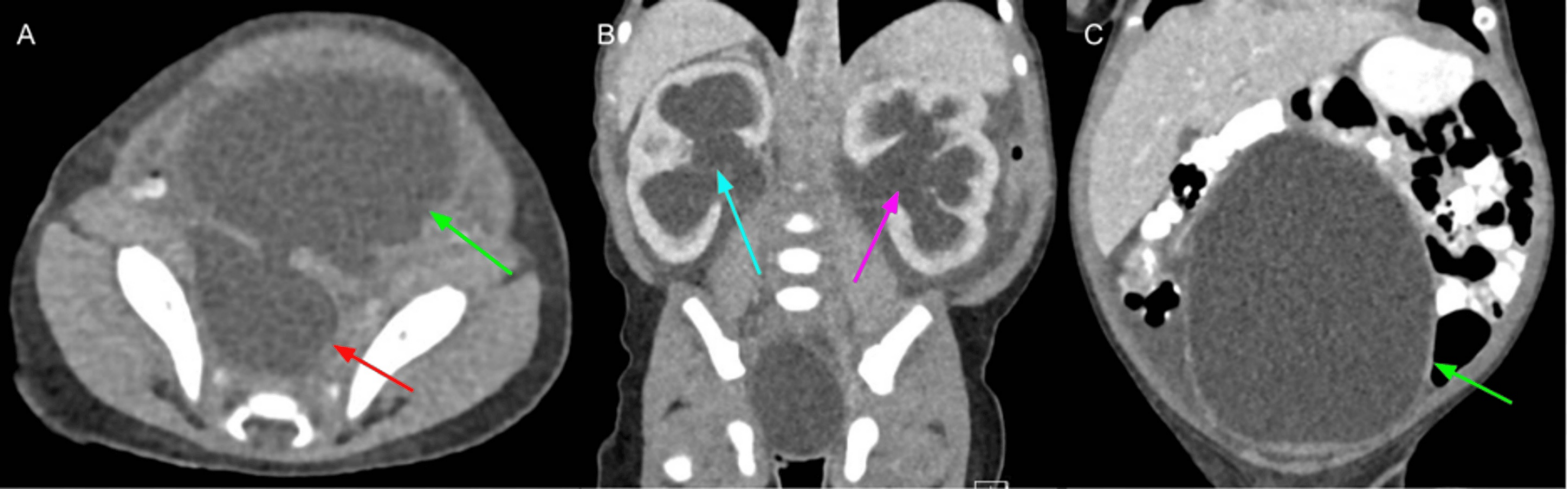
Computed tomography of the abdomen and pelvis at presentation (A) Transverse view demonstrating a distended bladder (green arrow) and bladder diverticulum (red arrow). (B) Coronal view demonstrating hydronephrosis of the right kidney (blue arrow) and hydronephrosis of the left kidney (purple arrow). (C) Coronal view demonstrating a massively distended bladder (green arrow).
